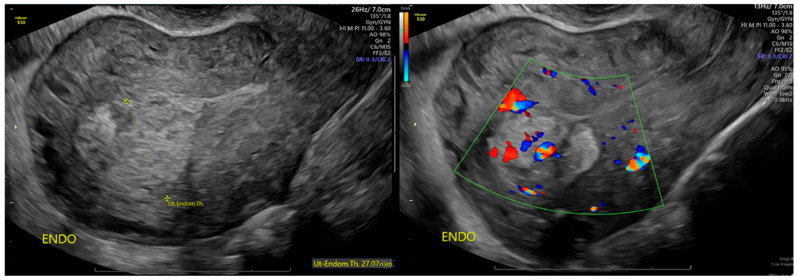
Ultrasound obtained after initial vacuum curettage showing heterogenous endometrium measuring 27 mm with Doppler ultrasound consistent with retained products of conception.
